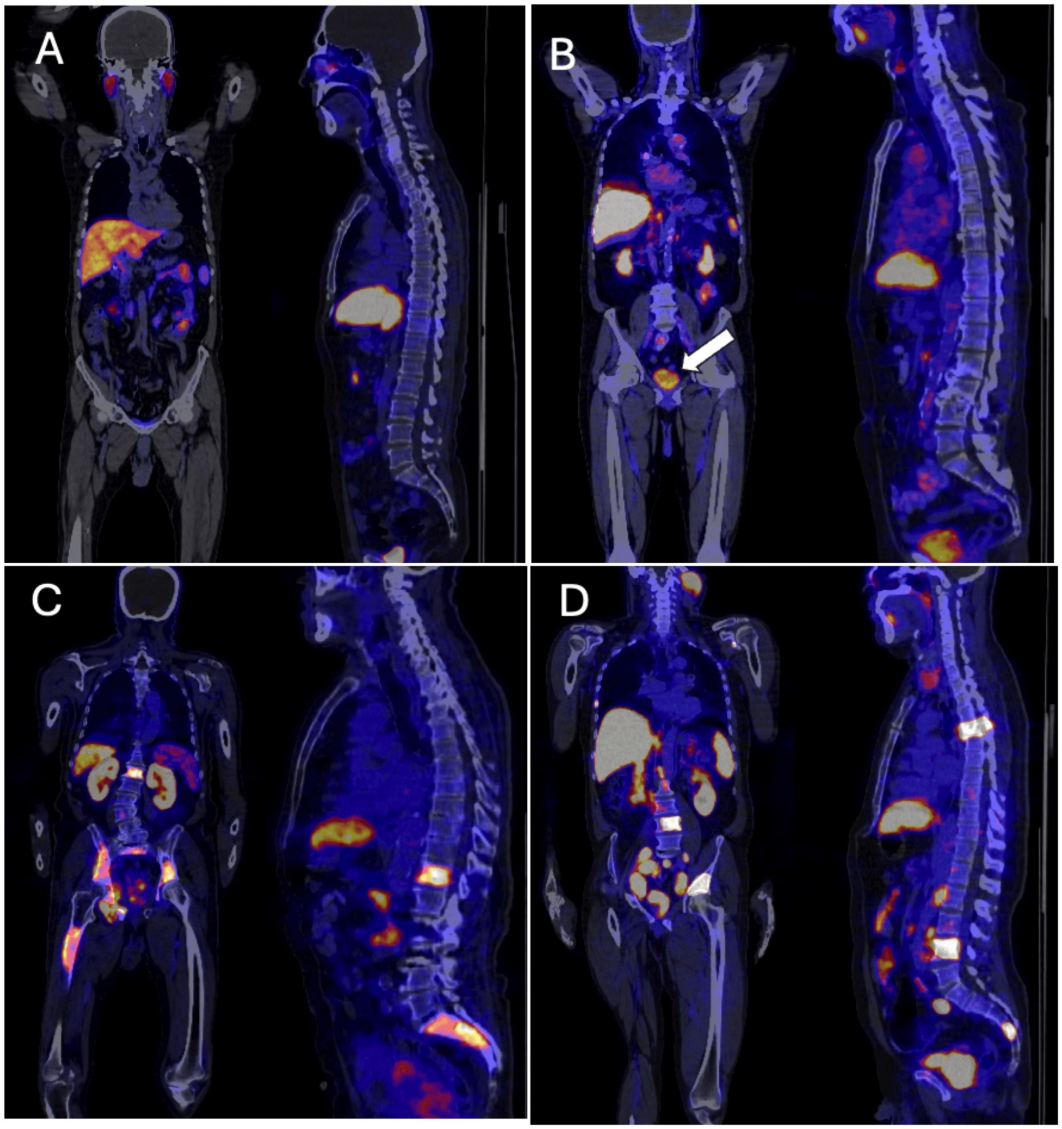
Representative PSMA PET/CT scan sample images from the cohort A. Negative PSMA PET/CT scan, indicating no detectable prostate cancer activity. B. Positive PSMA PET/CT scan confined to the prostate gland, showing localized cancer without evidence of metastasis. C. PSMA PET/CT scan revealing bone-only metastases, demonstrating PSMA uptake within the osseous structures, and DPSMA PET/CT scan displaying both bone metastases and lymph node involvement at multiple levels, indicating advanced metastatic disease with dissemination to the skeletal and lymphatic systems.
